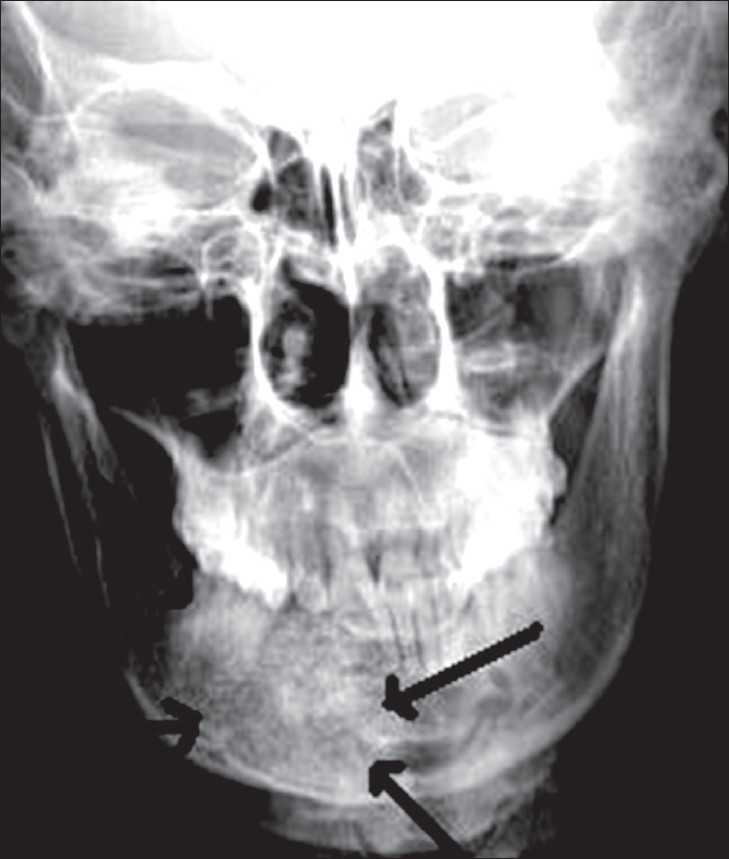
Radiograph of the mandible (PA view) shows a diffuse, radiodense lesion with a honeycomb appearance in the anterior mandible on the right, with lingual displacement of the adjacent teeth and possible extension across the symphysis
